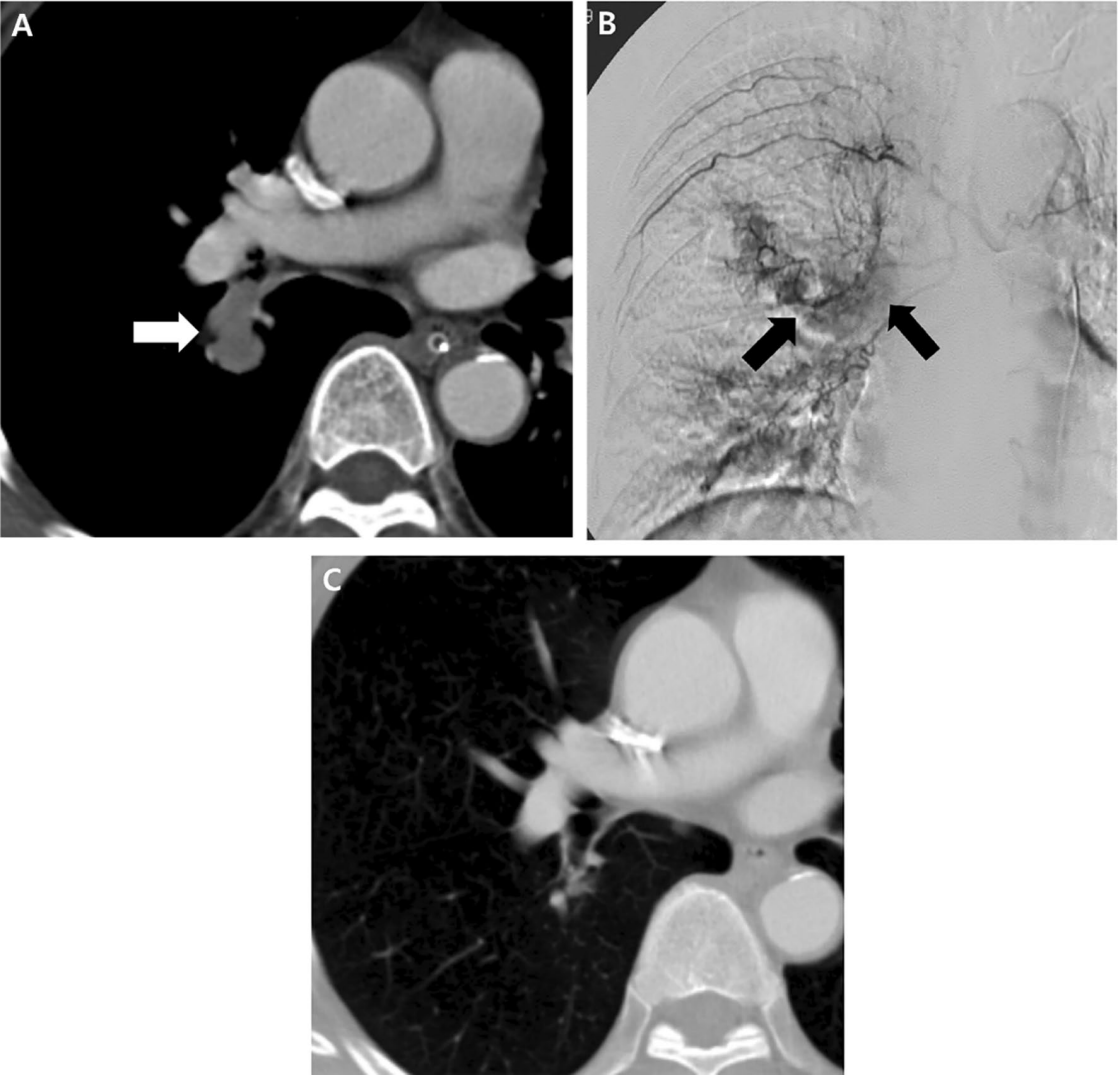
A 72-year-old man with pulmonary metastasis from HCC who presented with a moderate amount of hemoptysis. (A) Axial chest CT image before embolization shows an intraluminal soft-tissue mass in the proximal right lower lobe lobar bronchus (white arrow). (B) Selective right bronchointercostal trunk arteriography using a 5-F catheter shows multiple hypervascular tumor blushes along the bronchial artery. Note that a hypervascular tumor blush (black arrows) in the proximal right lower lobe lobar bronchus corresponds with endobronchial soft tissue mass in the axial CT image. Embolization of the right bronchial artery was successfully performed using gelfoam and a microcoil, and this patient showed complete resolution of hemoptysis following bronchial artery embolization. (C) Axial CT image 4 months after embolization of the bronchial artery shows a marked decrease in the size of the metastatic mass in the right lower lobe lobar bronchus.
